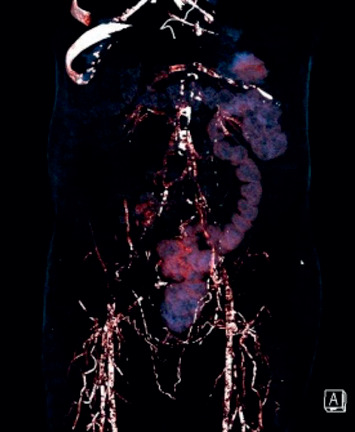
Volume-rendering technique image of the abdominal CT scan showing extensive vascular calcification.
